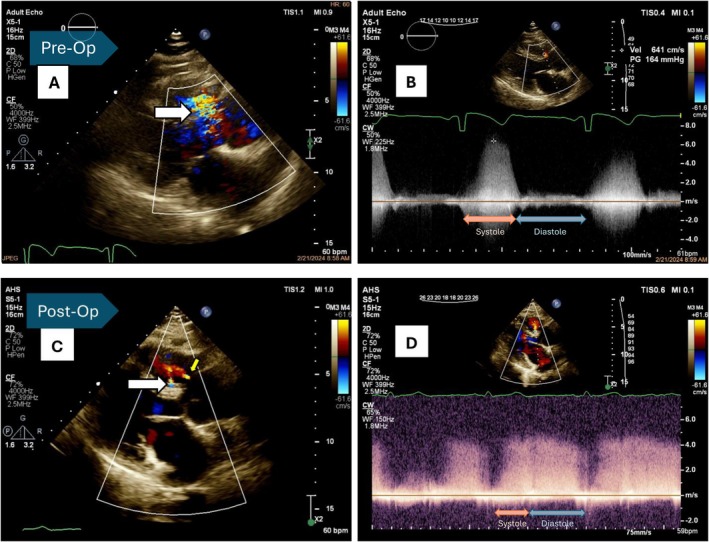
Transthoracic echocardiography; pre‐operative (A, B) and post‐operative (C, D). (A) *Pre‐operative* parasternal long‐axis view with color Doppler showing perimembranous VSD turbulent jet flow from interventricular septum, visible just below aortic valve. (B) Continuous‐wave Doppler tracing across the jet shows a predominantly systolic, high‐velocity flow pattern, consistent with VSD flow. (C) *Post‐operative* parasternal long‐axis view with color Doppler demonstrating the acquired coronary–cameral fistula, visualized as jet flow originating from the interventricular septum (lower than preoperative VSD jet flow visible in A) and directed toward the RVOT (white arrow). Pulmonary regurgitation diastolic flow is also observed in this view (yellow arrow). (D) Continuous‐wave Doppler tracing across the jet shows a continuous, predominantly diastolic high‐velocity flow pattern consistent with a coronary–cameral fistula.
